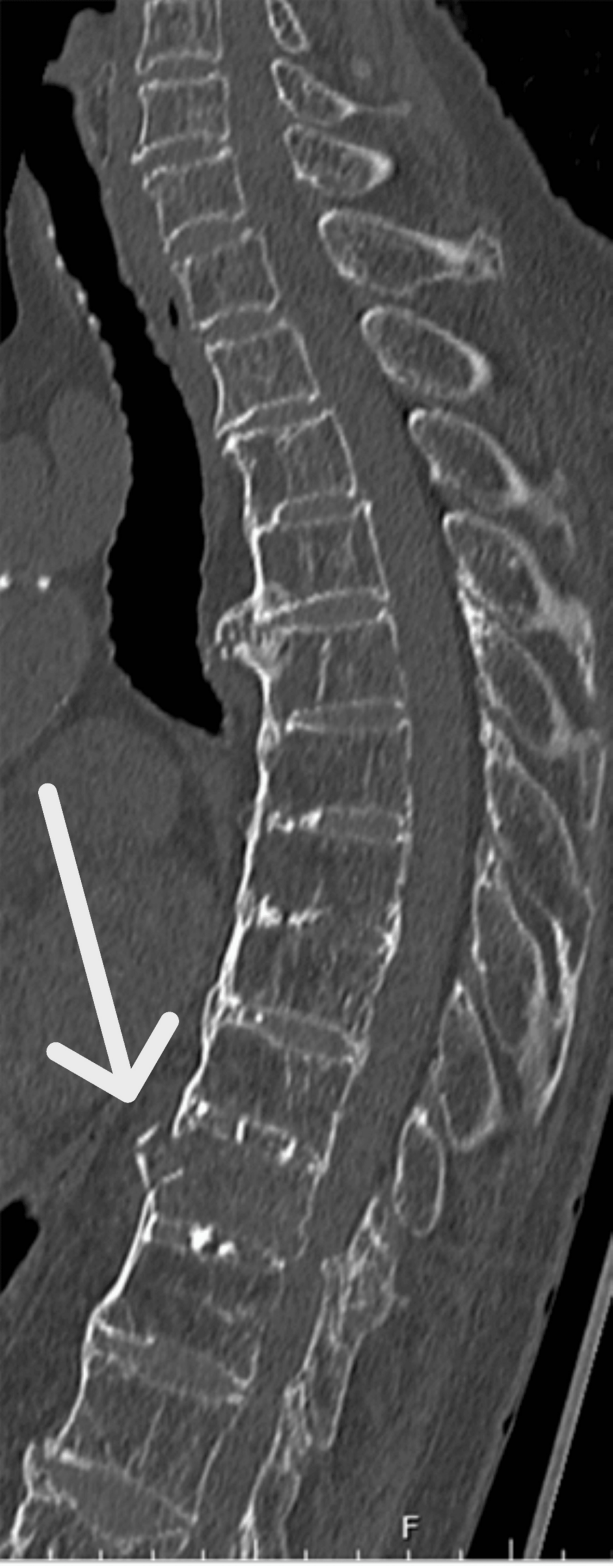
CT scans at diffuse idiopathic skeletal hyperostosis (DISH) spine diagnosis The sagittal CT depicted T10 anterior vertebral wall comminution and T10/11 canal stenosis due to the ossified yellow ligament.
